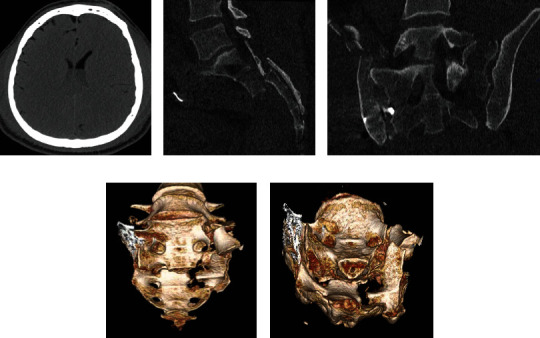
(a) Axial head CT scan indicating hyperdense fluid in the lateral ventricles. (b, c) Sagittal and coronal CT planes' indication on the spinopelvic dislocation and the unstable dorsal pelvic ring with a transiliosacral fracture on the right side and a transforaminal component on the left side. (d, e) 3D reconstruction of the CT scan showing the spinopelvic dislocation and sacral burst fracture (S2/3).
